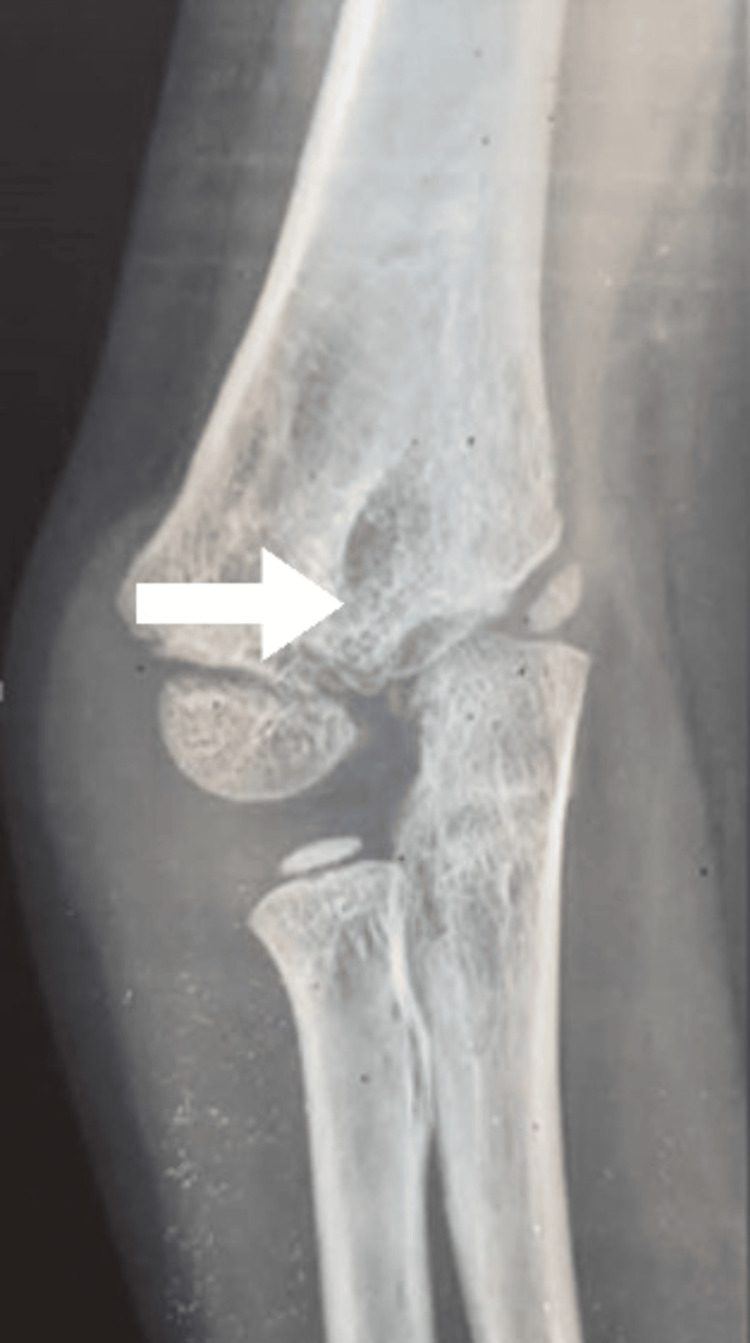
Anteroposterior X-ray of the right elbow at five-year follow-up Note the lucency involving the trochlea suggesting osteonecrosis (arrow)
